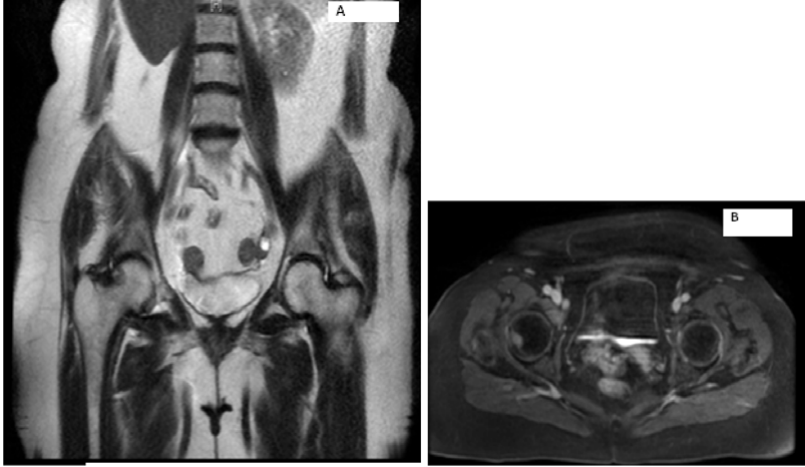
Magnetic resonance imaging of the abdomen showing metastasis to right hip.
